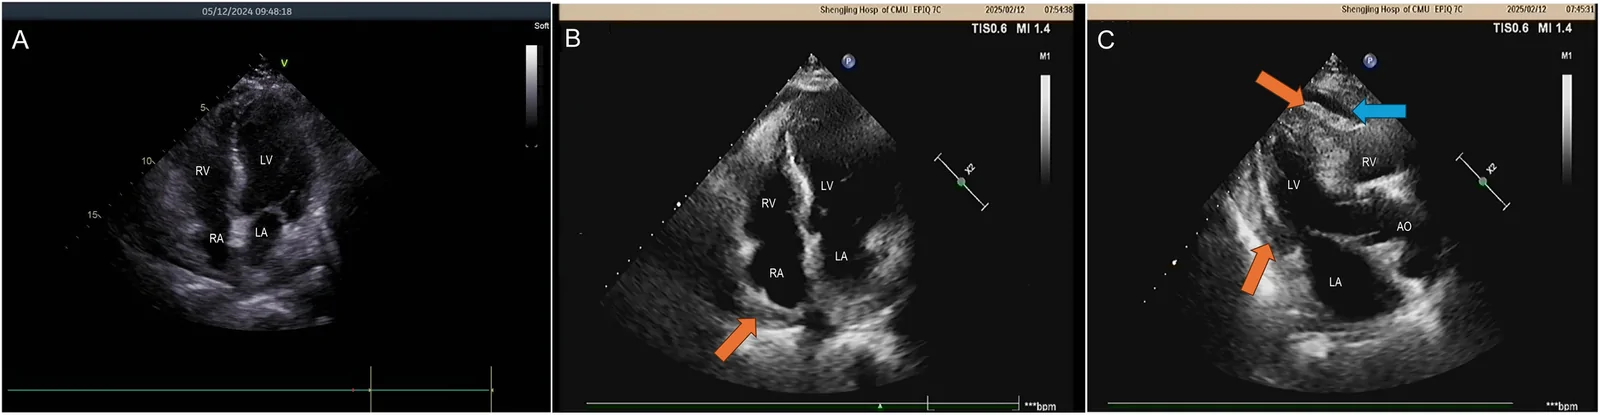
Transthoracic echocardiography before and after a percutaneous coronary intervention (PCI). (A) Before the PCI (5 December 2024): the pericardium is not thickened and shows no adhesion. (B,C) Current admission (12 February 2025): thickening of the visceral and parietal pericardium over the mid-to-lower left ventricle and the roof of the right atrium, with a hypoechoic layer within the pericardial space and focal visceral–parietal pericardial adhesion; a small pericardial effusion is seen elsewhere. The orange arrows indicate pericardial thickening; the blue arrows indicate the newly detected effusion. LV, left ventricle; RV, right ventricle; LA, left atrium; RA, right atrium; AO, aorta.
